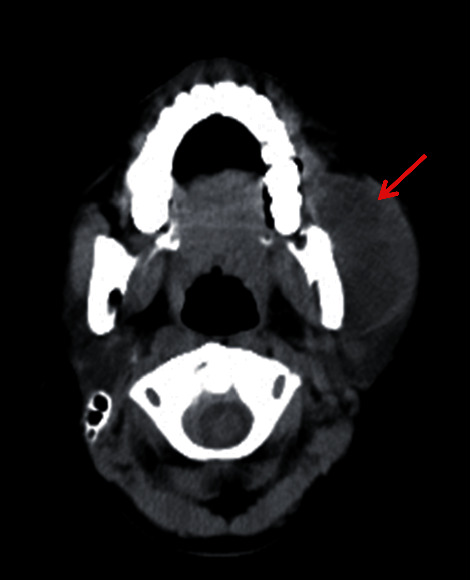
CT scan neck showing parotid abscess.
